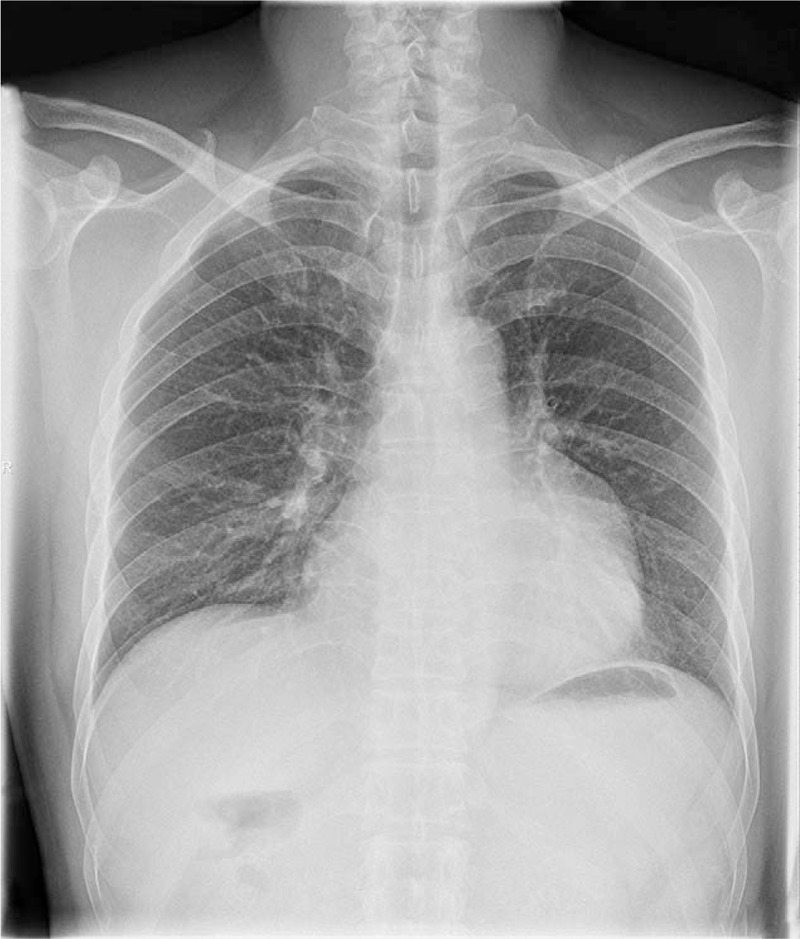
The posteroanterior chest x-ray demonstrating abnormal left heart border.
